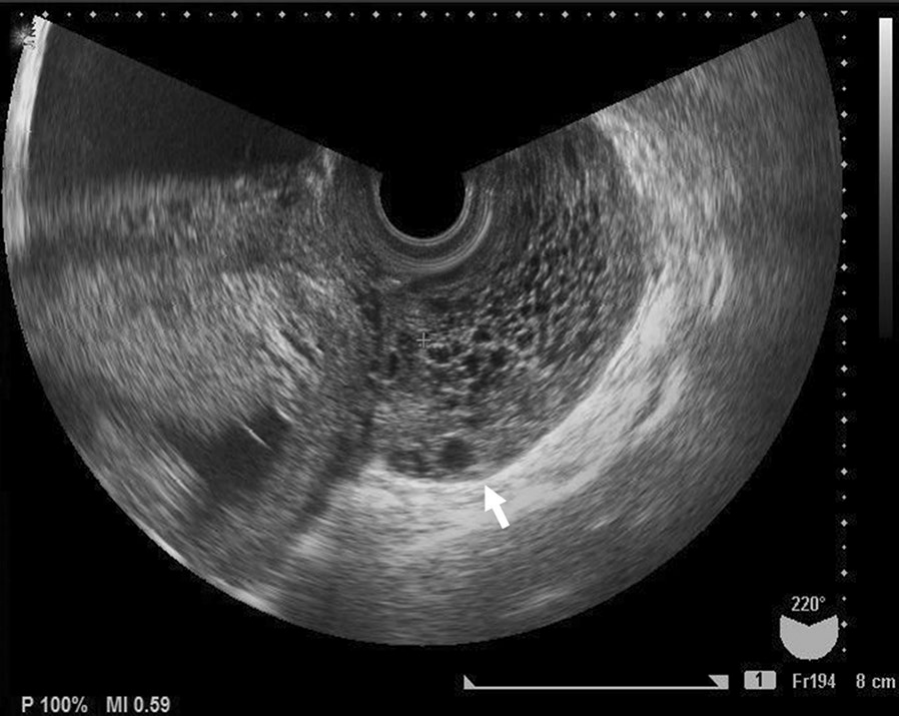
Transvaginal ultrasonography shows a solid mass (*arrow*) with hypoechogenic cysts in the Douglas’ pouch
